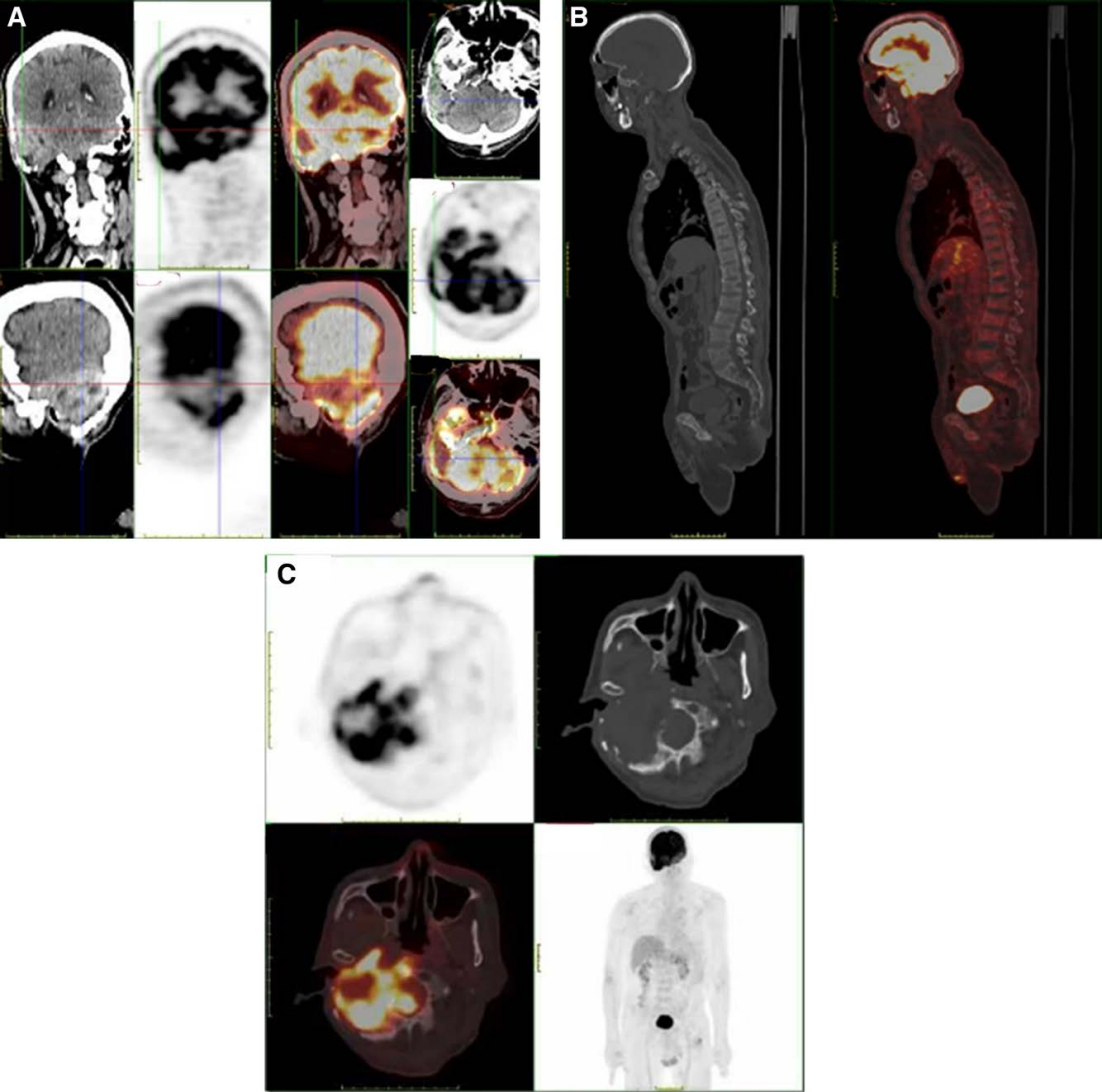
18F-FDG PET/CT (A and B) and 18F-OC PET/CT (C) revealed a mixed density mass in the right temporo-occipital region, with compression and displacement of the surrounding brain parenchyma, bone destruction of the right sphenoid, temporal bone and part of the occipital bone, and radioactive distribution was unevenly increased (18F-FDG PET/CT: SUVmax = 6.8, 18F-OC PET/CT: SUVmax = 10.9). Thoracic deformity was seen, with multiple ribs substance disorganization and osteotylus formation, and radioactivity distribution was mildly increased (18F-FDG PET/CT: SUVmax = 1.1, 18F-OC PET/CT: SUVmax = 2.0). Striped low-density shadows were seen in the bilateral proximal femur, and the radioactivity distribution of the left proximal femur was abnormally high (18F-FDG PET/CT: SUVmax = 7.1, 18F-OC PET/CT: SUVmax = 4.1). Decreased bone density in the skull, spine, pelvis, and extremities, multiple osteophyte formations in the lumbar spine, with no obvious abnormalities in radioactive distribution. 18F-FDG PET/CT results suggested that the right temporo-occipital mass after radiotherapy, inhomogeneous increase in metabolism, and the temporo-occipital tumor was considered the responsible lesion. 18F-OC PET/CT results suggested that the right temporo-occipital lesion had inhomogeneous high expression of octreotide, and the high probability of PMT. 18F-FDG = 2-deoxy-2-[fluorine-18] fluoro-d-glucose, 18F-OC = 18F-AlF-NOTA-octreotide, PET/CT = positron emission tomography/computed tomography, PMT = phosphaturic mesenchymal tumor.
